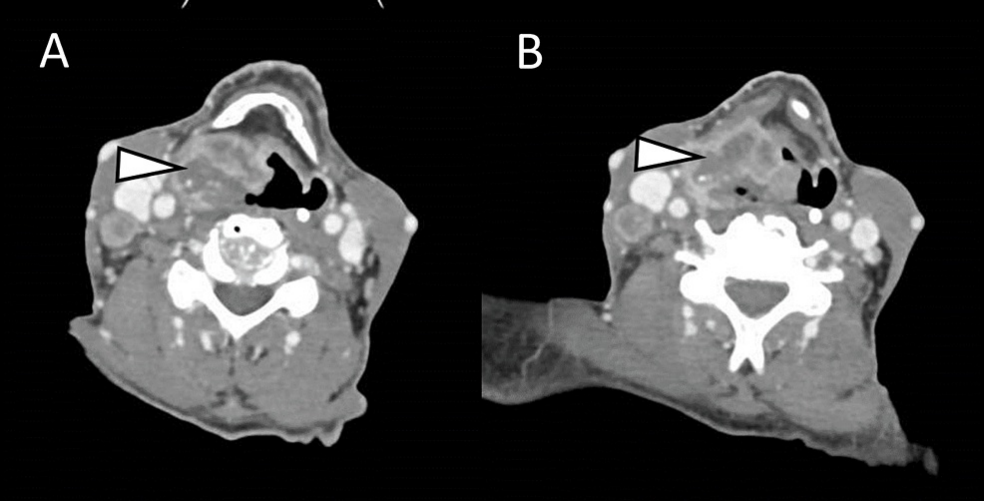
Contrast-enhanced computed tomography of the neck, thorax, abdomen, and pelvis Expansive infiltrative lesion localized at the right piriform recess and epiglottis, with a diameter of 4.5 cm and an ulcerated center. The lesion extends inferiorly to the right supraglottic fat tissue above the cricoid cartilage and the arytenoids, crossing over the midline. Arrowheads point to the laryngeal carcinoma.
